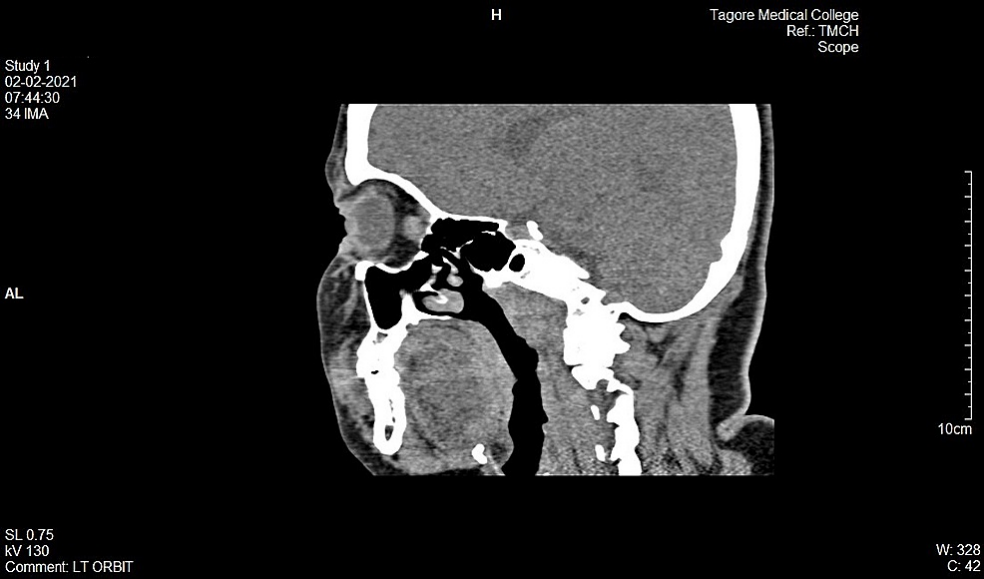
Postop 22-month CT scan (sagittal): Posteriorly the graft seated in a sound bone
